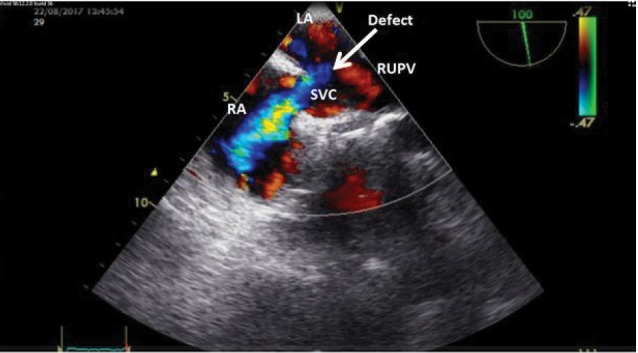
Patient received intravenous vancomycin, metronidazole and ceftriaxone for a total of 6 weeks and was cured. His headache was gradually resolved. At the time of discharge from the hospital, no lesion was detected in brain CT-scan. No physical and mental symptom was reported in 6 months follow up. In the control MRI which was provided 6 months after surgery no positive finding except mild arrested hydrocephaly was reported.
